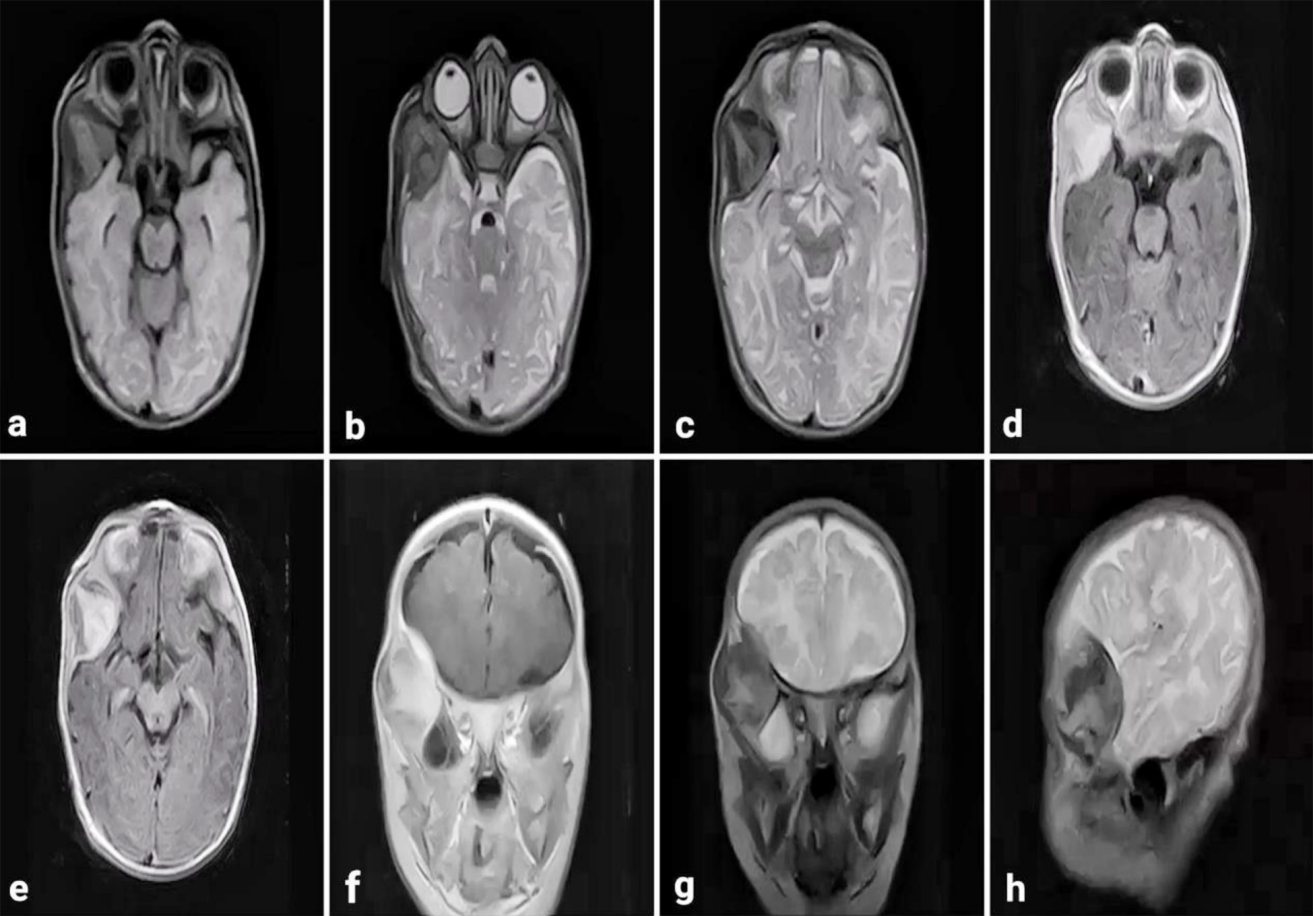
(a) MRI brain T1-weighted axial view showing a hypointense extra-axial right temporal lesion. (b & c) T2-weighted axial view showing a similar lesion. (d & e) T1-weighted with contrast axial view showing contrast enhancement of the lesion and dural attachment. (f) T1-weighted with contrast coronal view also showing contrast enhancement. (g) T2-weighted coronal view showing hypointense lesion in right temporal base. (h) T2-weighted sagittal view of the same lesion.
